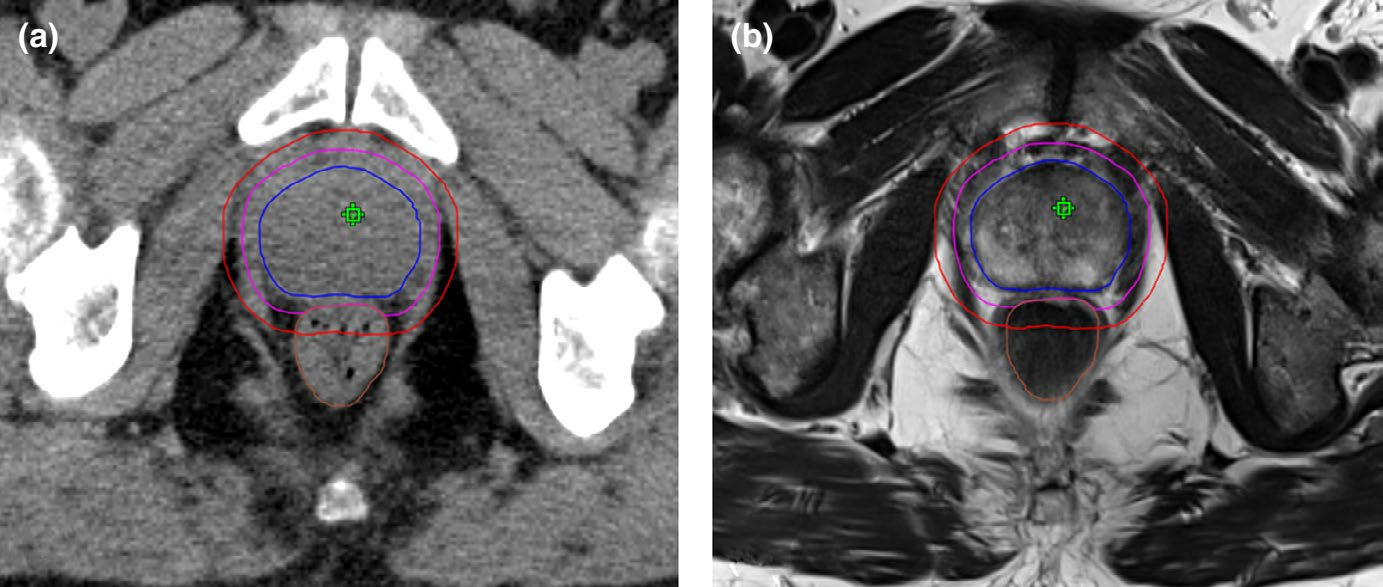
Target volume contours. Shown are the target volume contours of the CTV_P‐SV_ (blue), the high dose PTV_Boost_ (pink) and low dose PTV (red) in (a) the planning CT and (b) the planning MRI T2 sequence. The isocenter is marked in green. The low dose PTV was created by extending the CTV_P+SV_ (prostate with seminal vesicles) by 10 mm in all but dorsally directions, where a 7 mm margin was applied. The high dose PTV_Boost_ was created by extending CTV_P‐SV_ (prostate with only the base of the seminal vesicles) by 5 mm in all directions with avoidance of the organ at risk rectum
